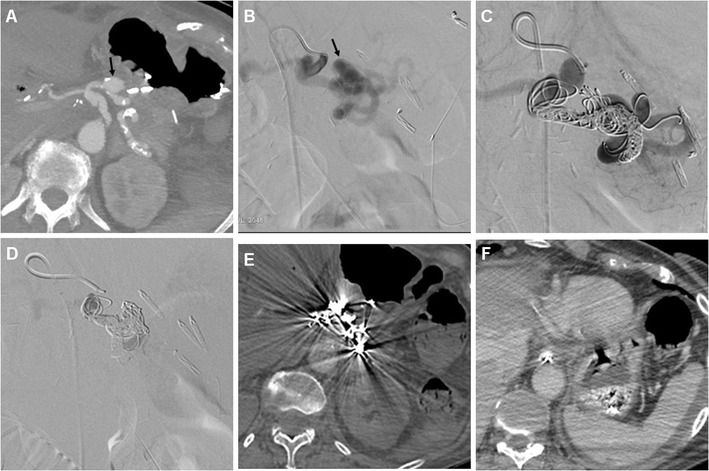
Endovascular treatment of postsurgical pseudoaneurysm in a 77-year-old woman. Three days after PD for pancreatic head adenocarcinoma, emergency CT was performed to investigate abdominal pain, blood from surgical drainage and dropping haemoglobin (>3 g/dL within 12 h). The pseudoaneurysm (*black arrow*) of the splenic artery depicted by MIP CT reconstruction (a) was confirmed angiographically (b). Embolisation was performed with a “sandwich technique”, placing coils both distally and proximally to the pseudoaneurysm, plus 0.2 ml of glue, due to persistent flow through the coils (c). Final angiogram (d) confirmed complete exclusion of the pseudoaneurysm. Follow-up CT confirmed successfully treated pseudoaneurysm (e) and normal perfusion of the spleen (f) supplied by collaterals
